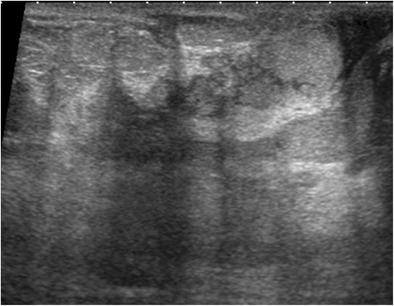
Abscess. A 42-year-old woman presenting with enlargement of the left breast for 1 month with pain and erythema related to mastitis of the left breast. Ultrasound scan of the left breast in the same patient revealing a mixed echogenicity, globally hyperechoic abscess
